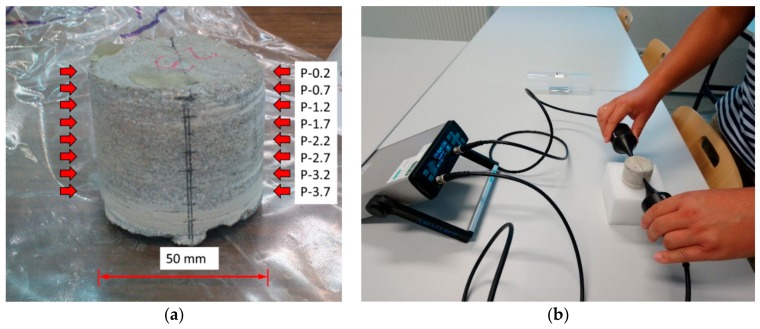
The ultrasonic method: (a) the arrangement of measuring points on a core sample for testing the longitudinal velocity of the ultrasonic wave *c*_L_ along the thickness of the cement mortar used to make the overlay; (b) the view of the core sample during the test.
